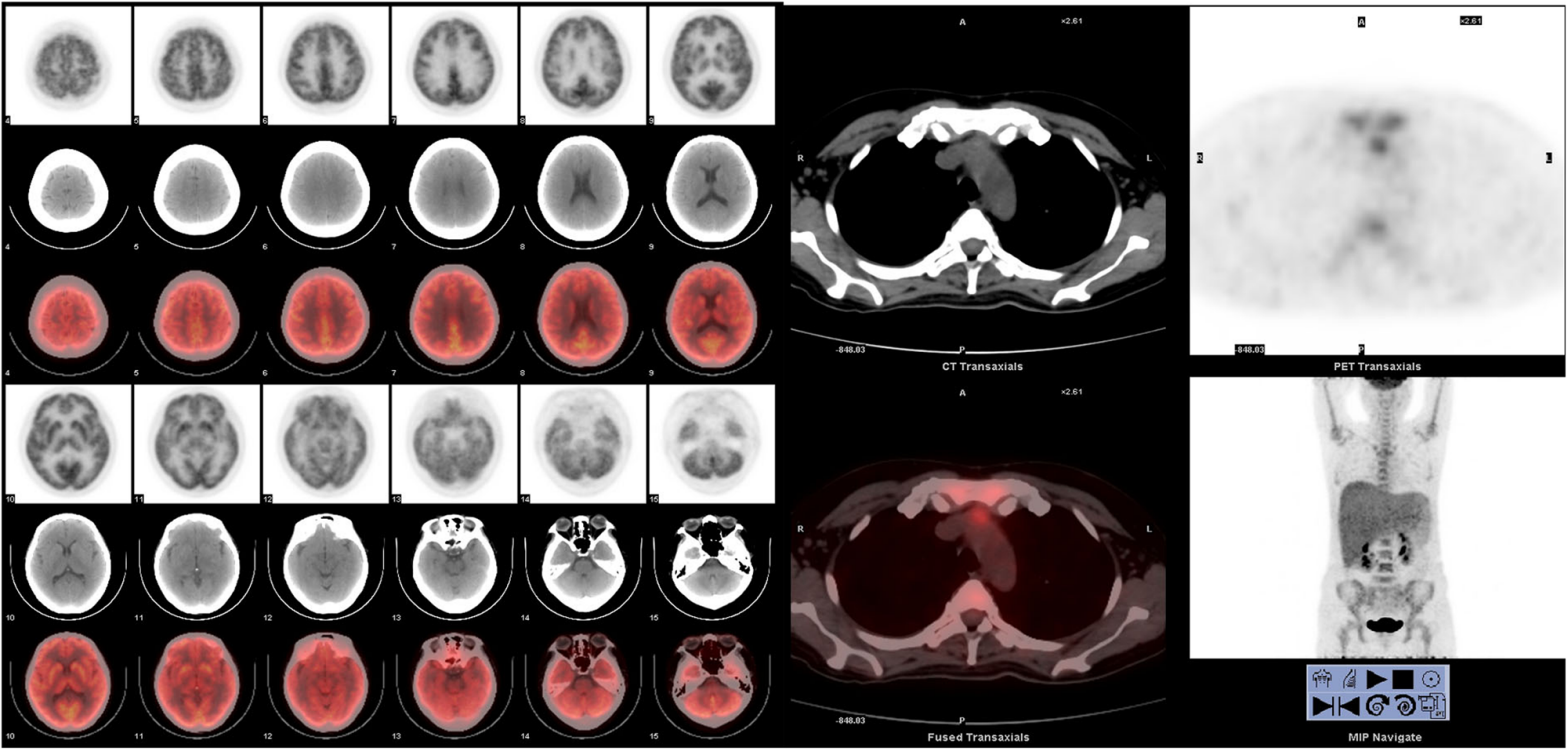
The patient’s first PET-CT report indicated that the systemic primary lesion was found before the mediastinum, and no abnormal hypermetabolism was found in the brain.
